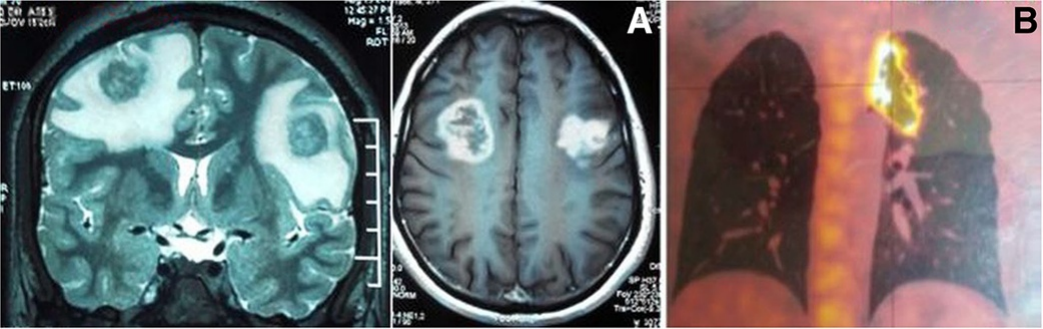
Non contrast computed tomograph of brain and whole body positron emission tomography scan.
